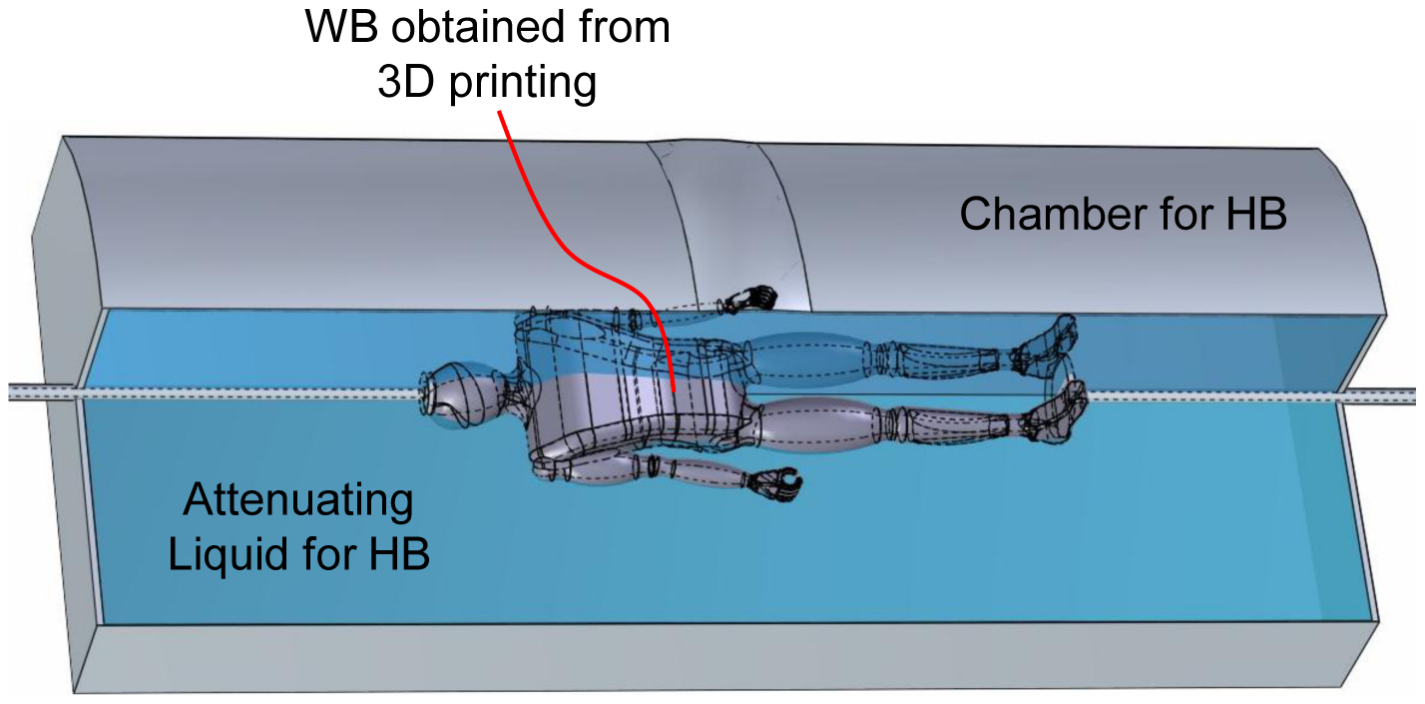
Dynamic bowtie for full body helical CT, in which the WB is rapidly prototyped according to an individualized patient contour obtained from surface scanning.
